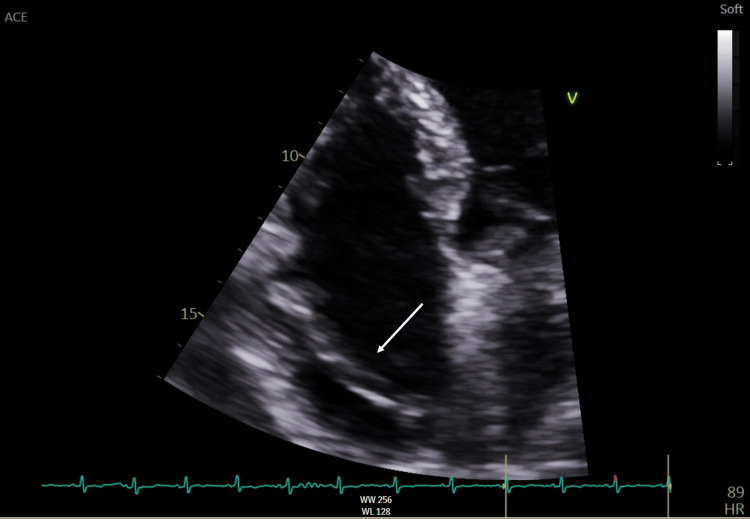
Transthoracic echocardiogram (TTE) identifying a large, highly mobile linear structure in the right atrium (arrow)
